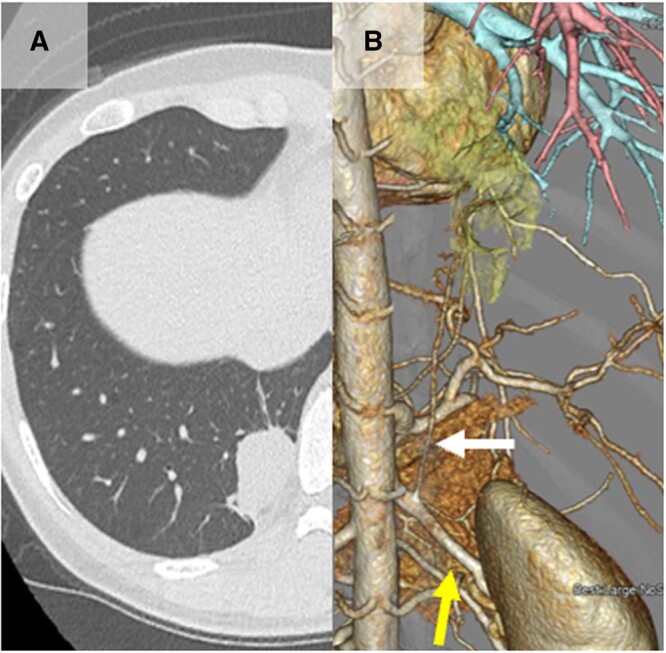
(A) Sequestered lung in dorsobasal segment (S10) of the right lower lobe as a isodensity mass on computed tomography. (B) Three-dimensional computed tomography showing an aberrant artery (white arrow) arising from the right renal artery (yellow arrow) (A color version of this figure appears in the online version of this article).
